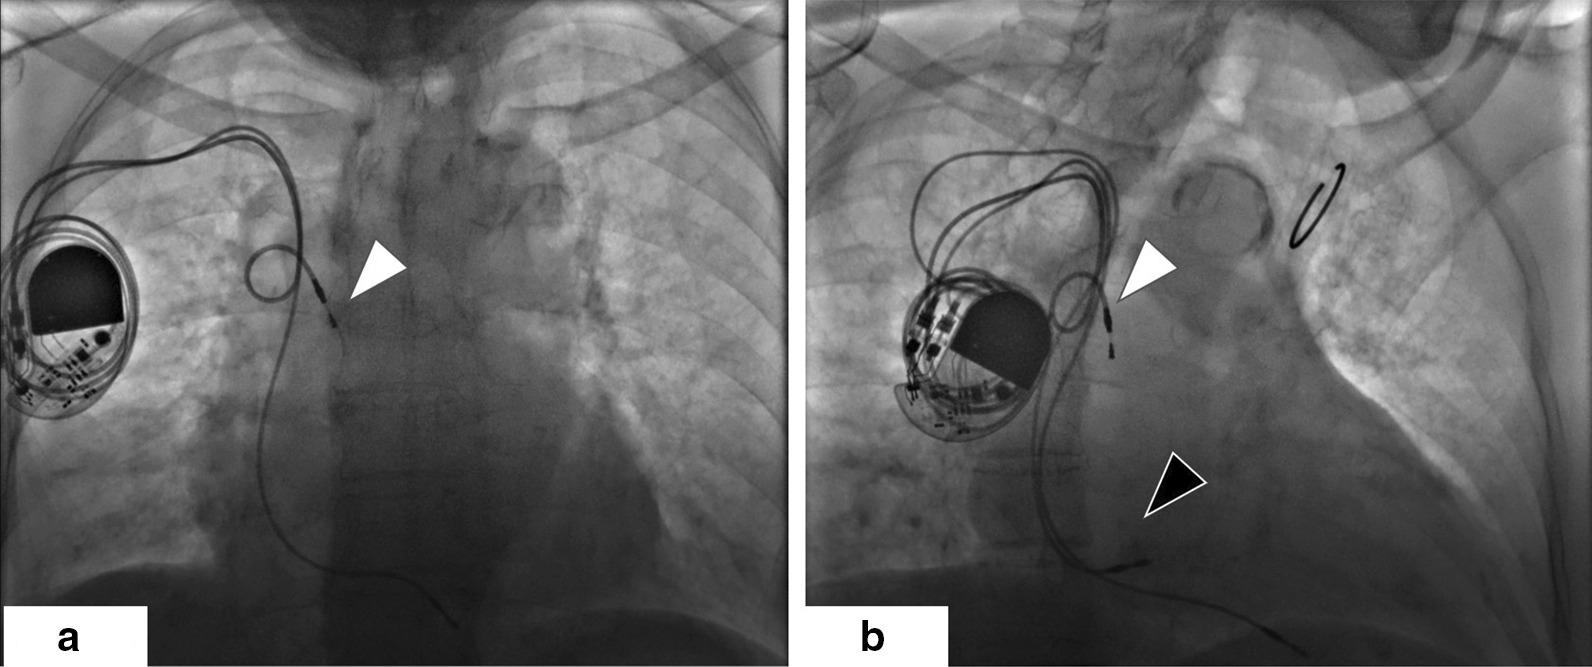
Anteroposterior projected radiograph of the patient before (a) and after (b) new atrial lead implantation. a The dislodged atrial lead in the superior vena cava, indicated by the white arrow. b The new atrial lead implanted in the right lower septum, indicated by the black arrow
